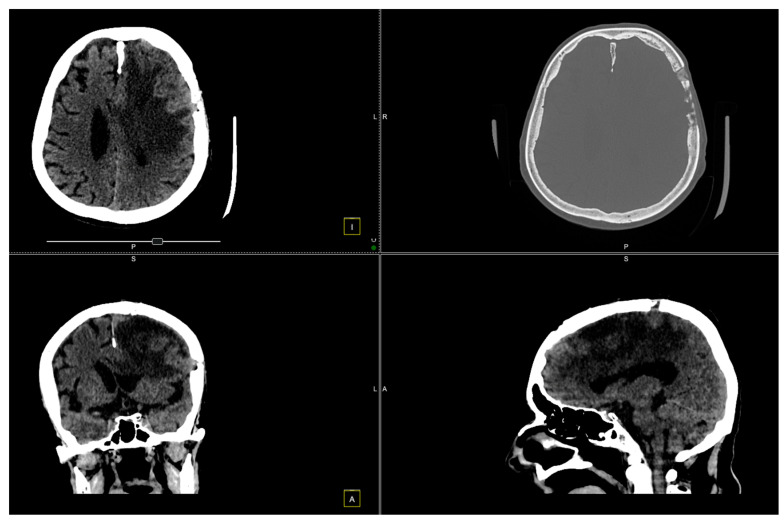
CT scan conducted 14 days after the surgery.
